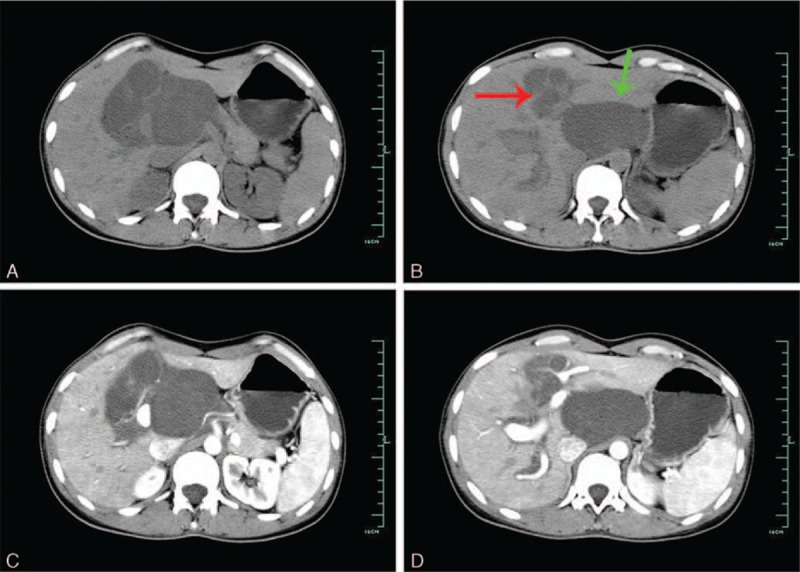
An unenhanced CT imaging showed multiple cystic lesions in the left liver (red arrow), as well as the space between left kidney and body of the stomach (green arrow) (A and B). On the contrast-enhanced CT, these lesions were not obviously enhanced (C and D). CT = computed tomography.
